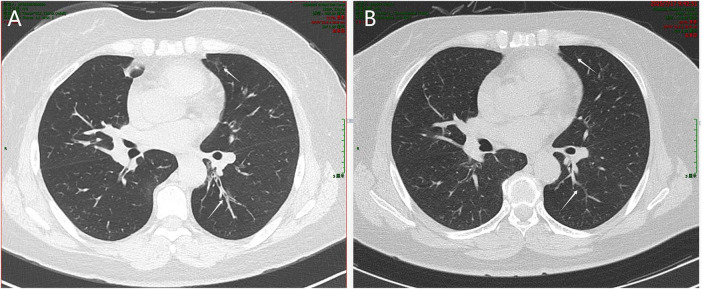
Comparison of chest CT findings before and after treatment. (A) Pretreatment chest CT (lung window, 2025-07-03) shows multiple bilateral patchy and nodular opacities with bronchiectasis (arrows). (B) Post-treatment chest CT (lung window, 2025-07-17) shows significant resolution of the pulmonary lesions and improvement of bronchiectasis (arrows).
